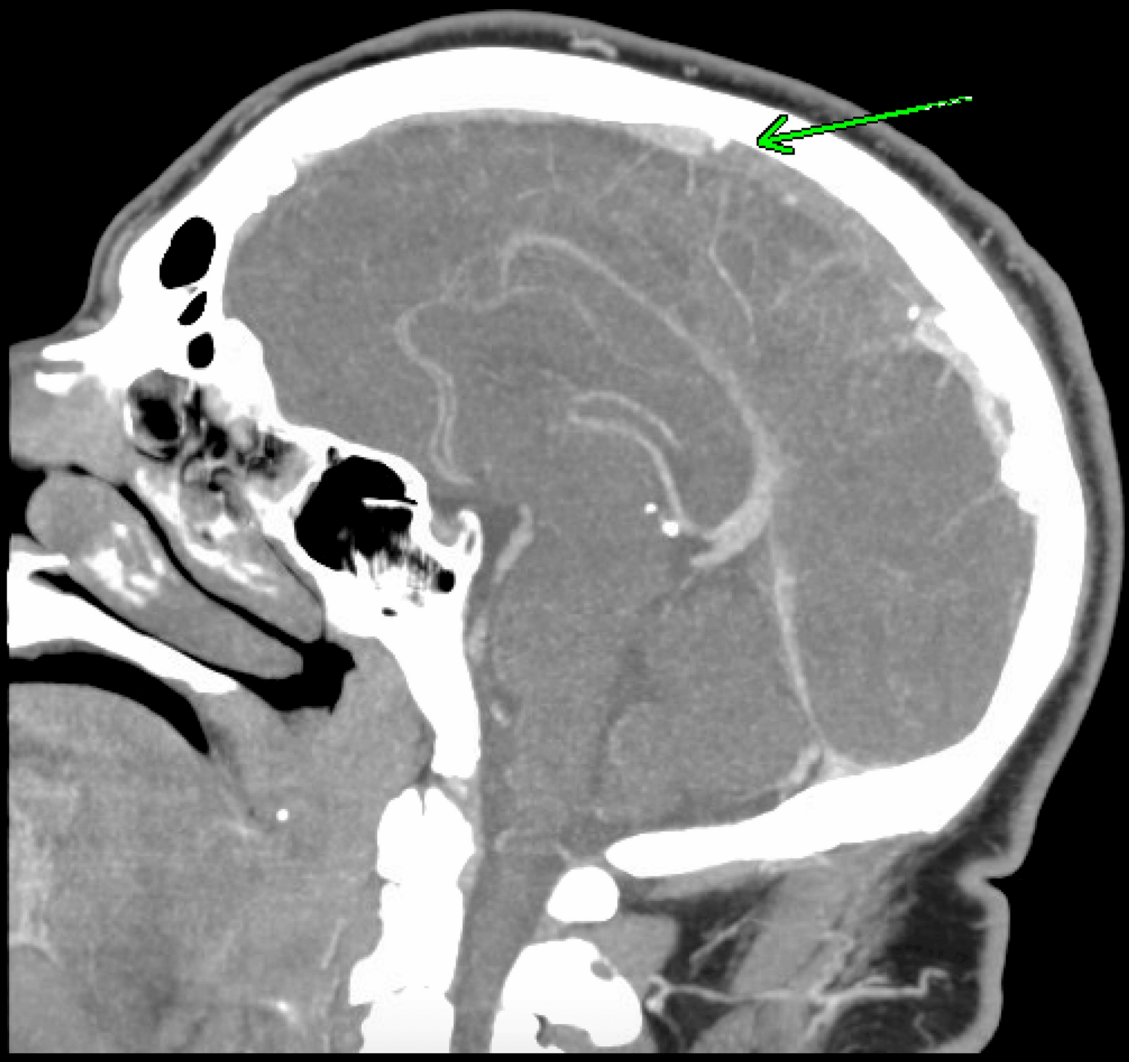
CT venogram of the head and neck showing thrombosis of the superior sagittal sinus (green arrow)
